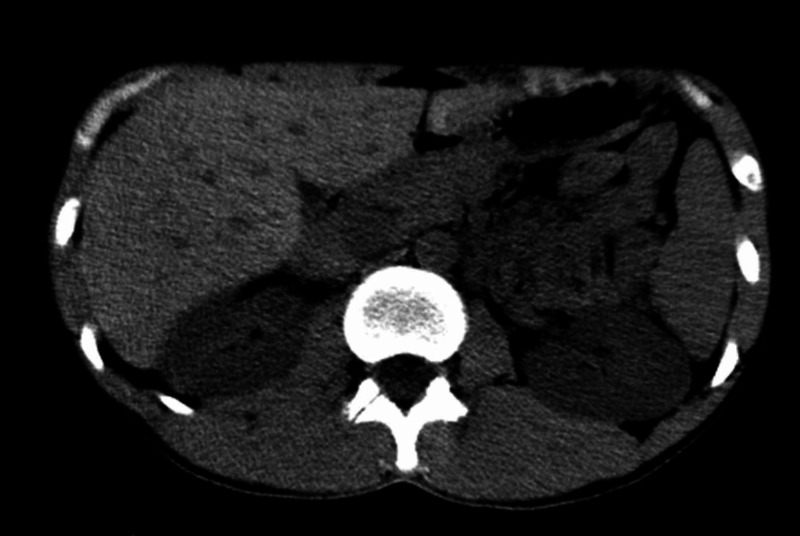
Axial image of CT scan of abdomen in abdominal window at the level of liver revealing no abnormality in visualized viscera CT, computerized tomography
